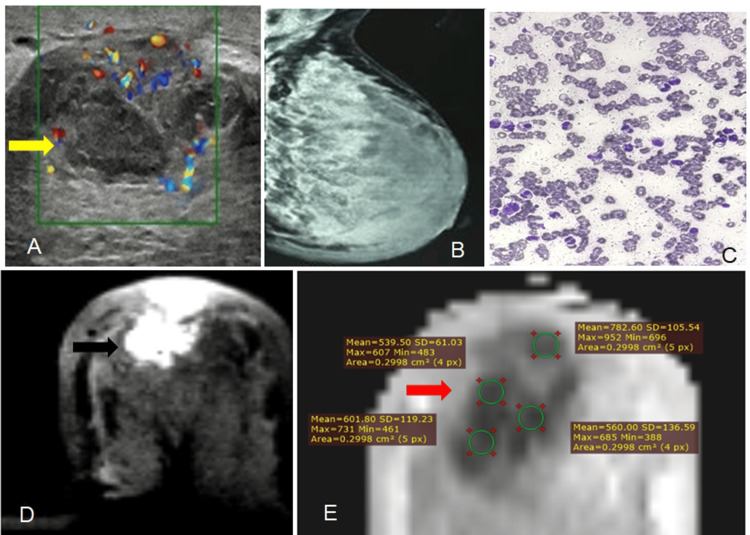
Mucinous Carcinoma (A) Ultrasound shows a heterogeneous, irregular lesion (white arrow) with indistinct margins and intralesional calcifications, categorized as BI-RADS IVc.
